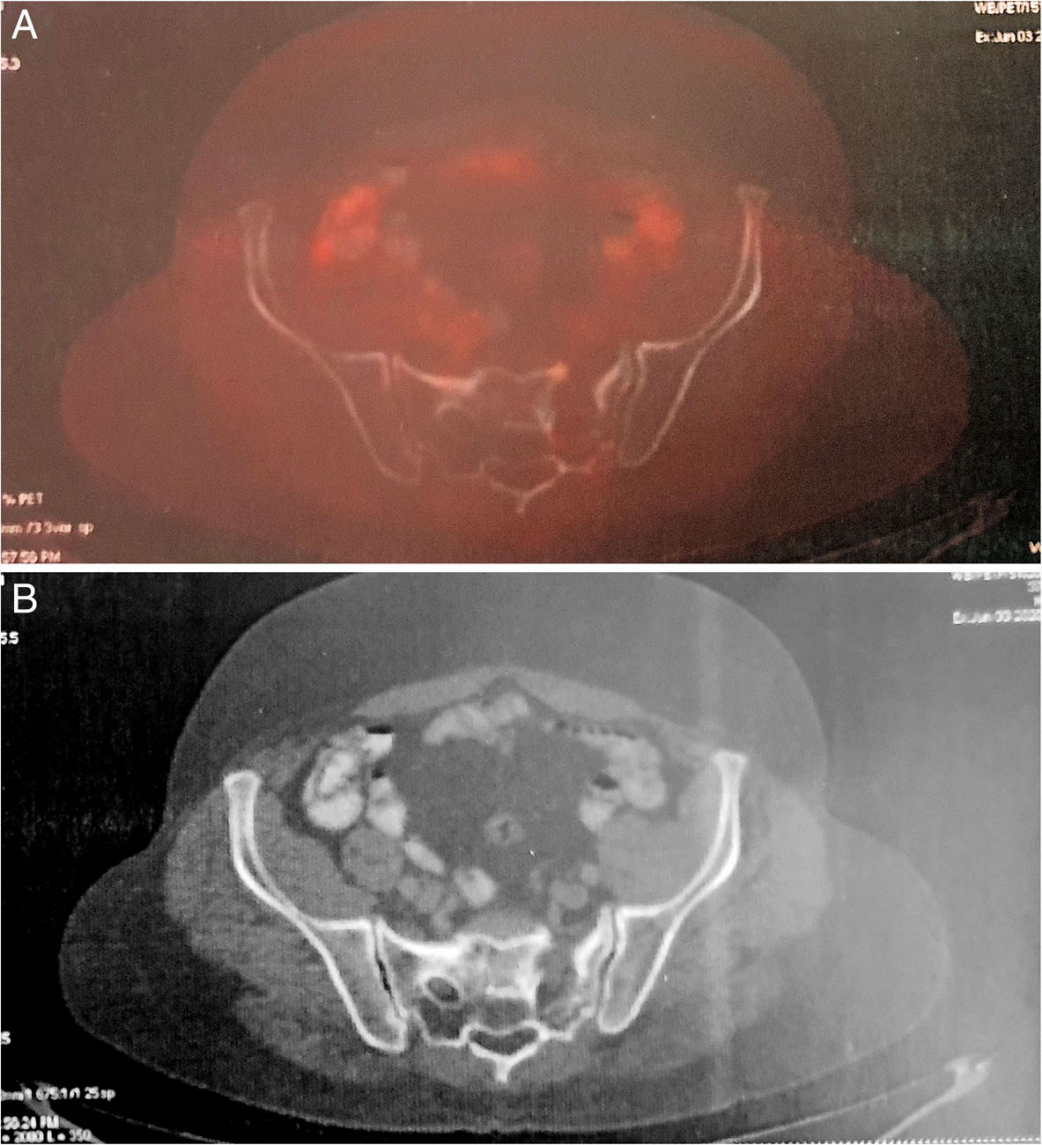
Follow-up FDG-PET in 2020. a Subtle sclerosis in the left sacral ala b with no FDG avid lesions
